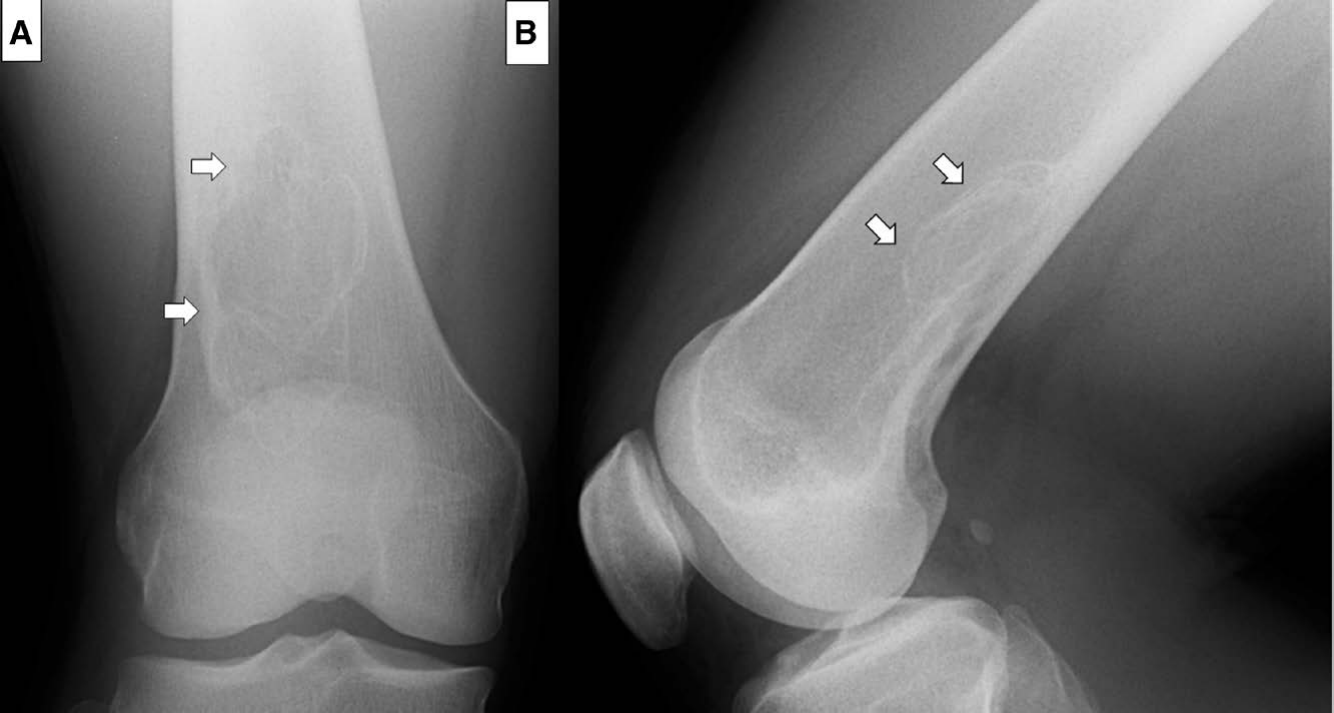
A 26-year-old male patient with a suspected malignant bone tumor diagnosis made by the primary physician. Anteroposterior (A) and lateral (B) views of the plain radiograph showing a large polycystic lesion with homogenous sclerosis (Ritschl stage D).
